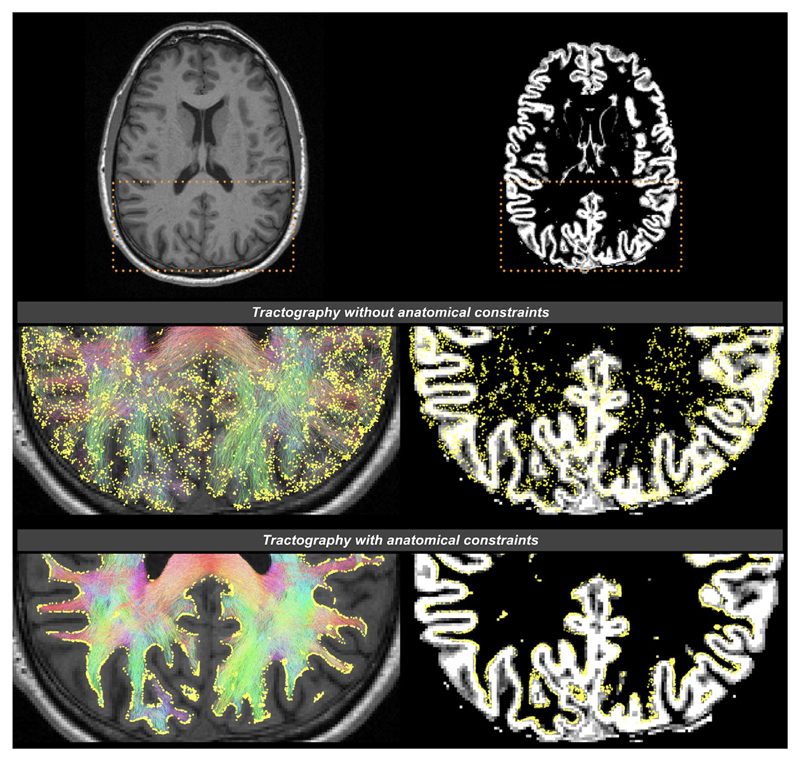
The effects of applying anatomical constraints on diffusion MRI streamlines tractography, shown on a transverse slice image of a human brain. The background images are a structural T1-weighted image on the left column, and the corresponding gray matter partial volume map following tissue segmentation on the right column. Streamlines are color-coded according to their orientations (red: left–right; green: anterior–posterior; blue: inferior–superior). The yellow spheres are the streamline endpoints. Top row: The dashed boxes indicate the zoomed brain areas shown in the middle and bottom rows. Middle row: Streamlines generated without anatomical priors; streamline endpoints distribute throughout the brain. Bottom row: Streamlines generated with anatomical priors; streamline endpoints only occur at the interface between GM and WM (demonstrated using Refs. 35, 42). It has been revealed that the considerable improvements in such streamline terminations provided by the use of anatomical constraints have significant influences on structural connectivity patterns and the outcomes of connectomic metrics.^43,44^
